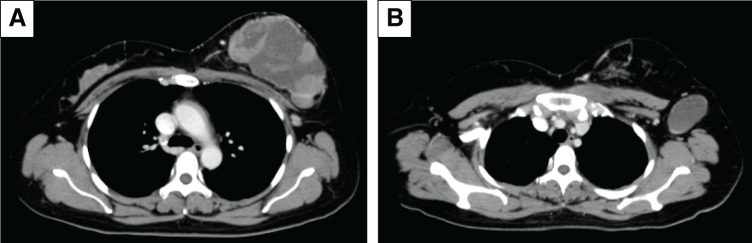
Contrast-enhanced CT. (A) The left breast tumor is composed of both liquid and solid components that are contrasted. (B) Cystic changes are observed in the axillary lymph nodes. These findings suggest a mixed internal tumor and lymph node metastasis.
